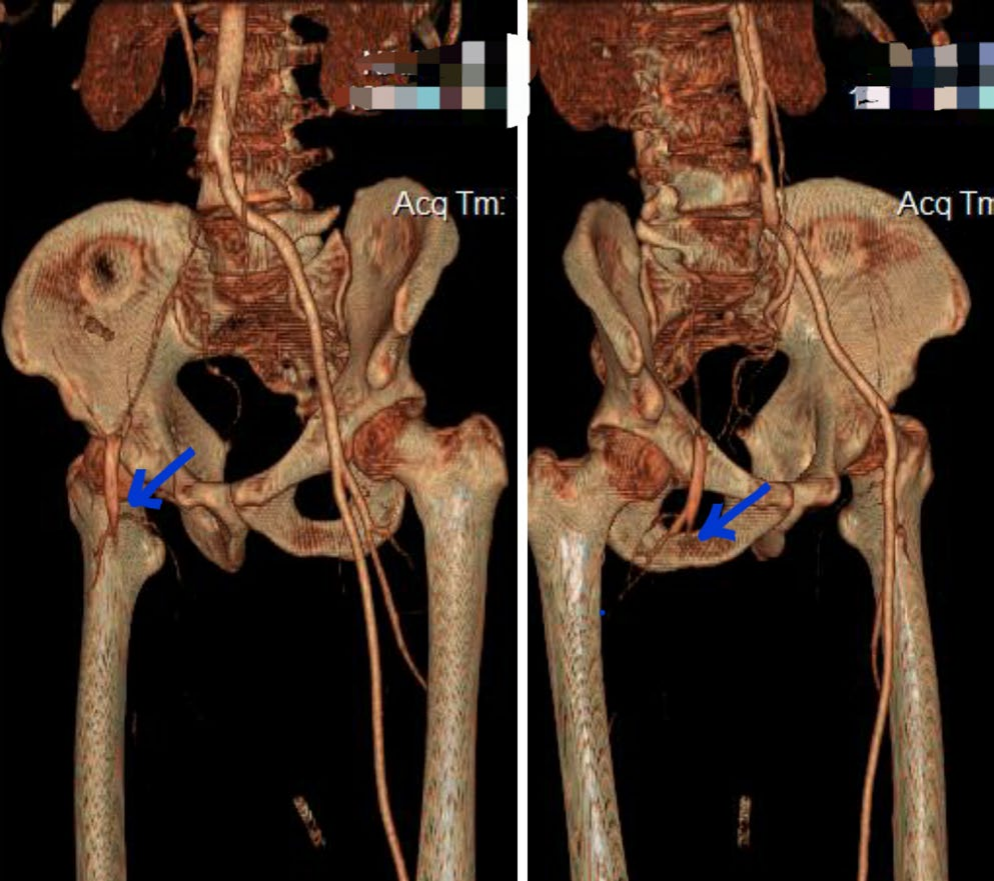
Reconstructed 3D CT angiography shows a cutoff distal to the common iliac artery (blue arrows)
